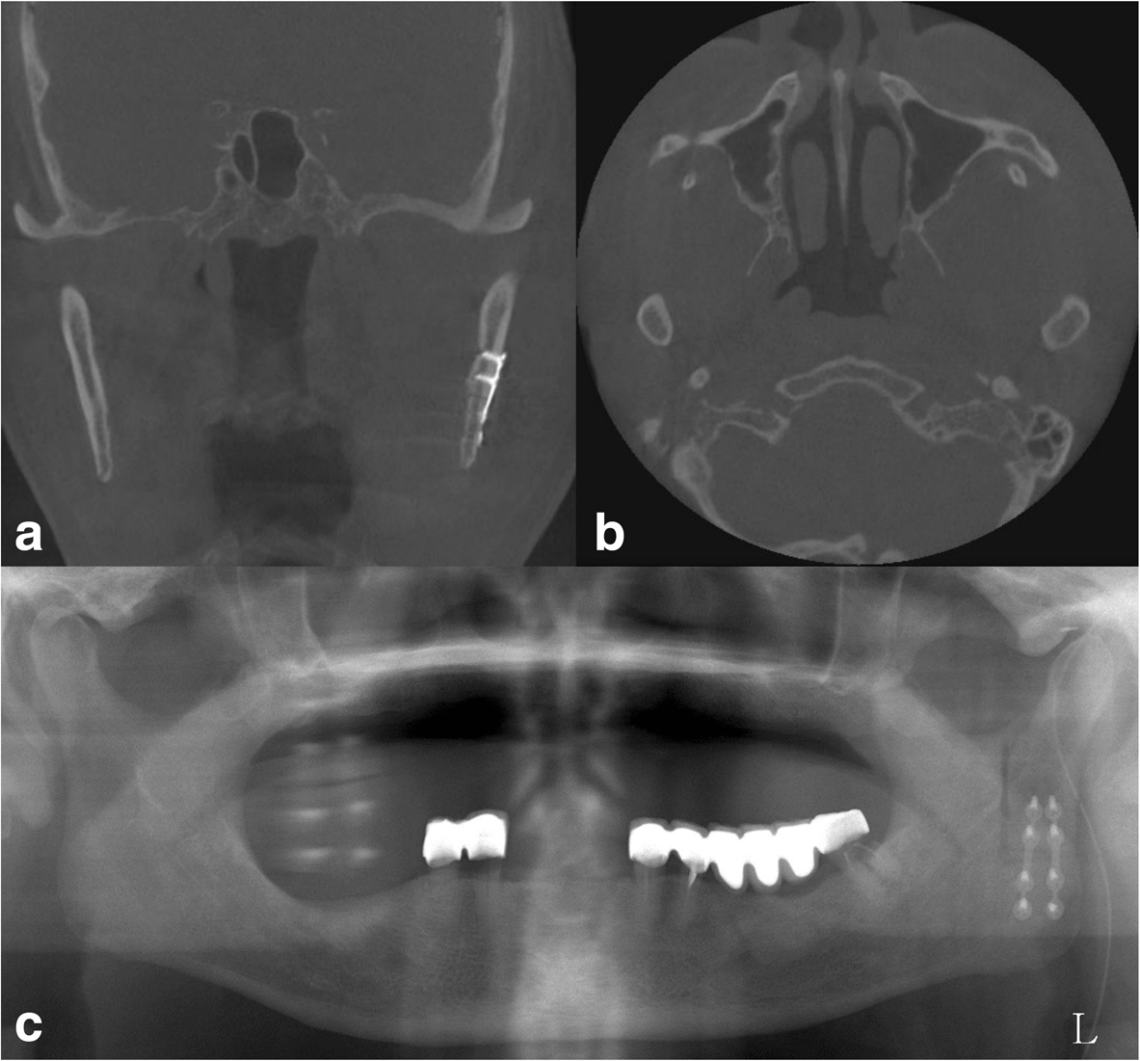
a Anatomical repositioning of the segmented condyle after mass excision and b complete excision of the mass from the infratemporal fossa in CBCT. c Panoramic view after operation
